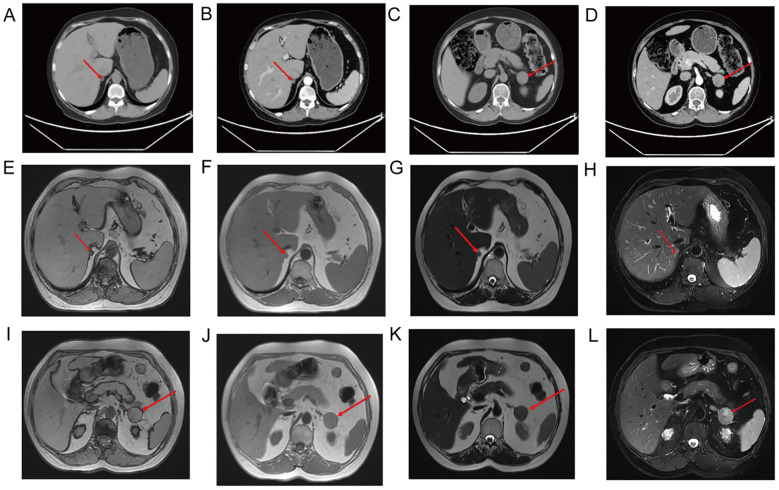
Multimodal imaging of the adrenal glands in Case 1. (A) Unenhanced CT demonstrating an 8 mm hypodense nodule in the right adrenal gland, with an attenuation value of −9 HU (arrow). (B) Contrast-enhanced CT demonstrating an 8 mm hypodense nodule in the right adrenal gland (arrow). (C) Unenhanced CT demonstrating an 28×27 mm hypodense nodule in the left adrenal gland, with an attenuation value of 26 HU (arrow). (D) Contrast-enhanced CT demonstrating an 28×27 mm hypodense nodule in the left adrenal gland (arrow). (E) T1-weighted out-of-phase MRI of the right adrenal gland. (F) T1-weighted in-phase MRI of the right adrenal gland. (G) T2-weighted MRI of the right adrenal gland. (H) Fat-suppressed T2-weighted MRI of the right adrenal gland. (I) T1-weighted out-of-phase MRI of the left adrenal gland. (J) T1-weighted in-phase MRI of the left adrenal gland. (K) T2-weighted MRI of the left adrenal gland. (L) Fat-suppressed T2-weighted MRI of the left adrenal gland. CT, computed tomography; MRI, magnetic resonance imaging; HU, hounsfield unit.
